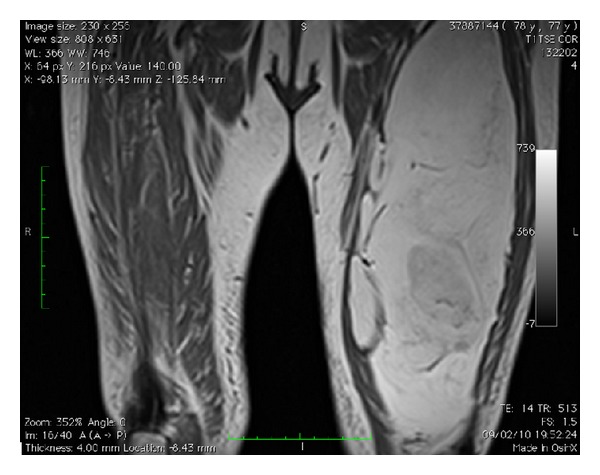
MRI of a lipomatous tumor in the anterior compartment of the thigh.
